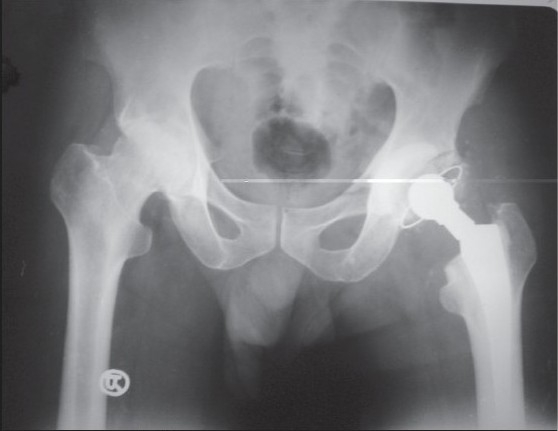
X-ray pelvis with both hips showing stage 1 – Muller
